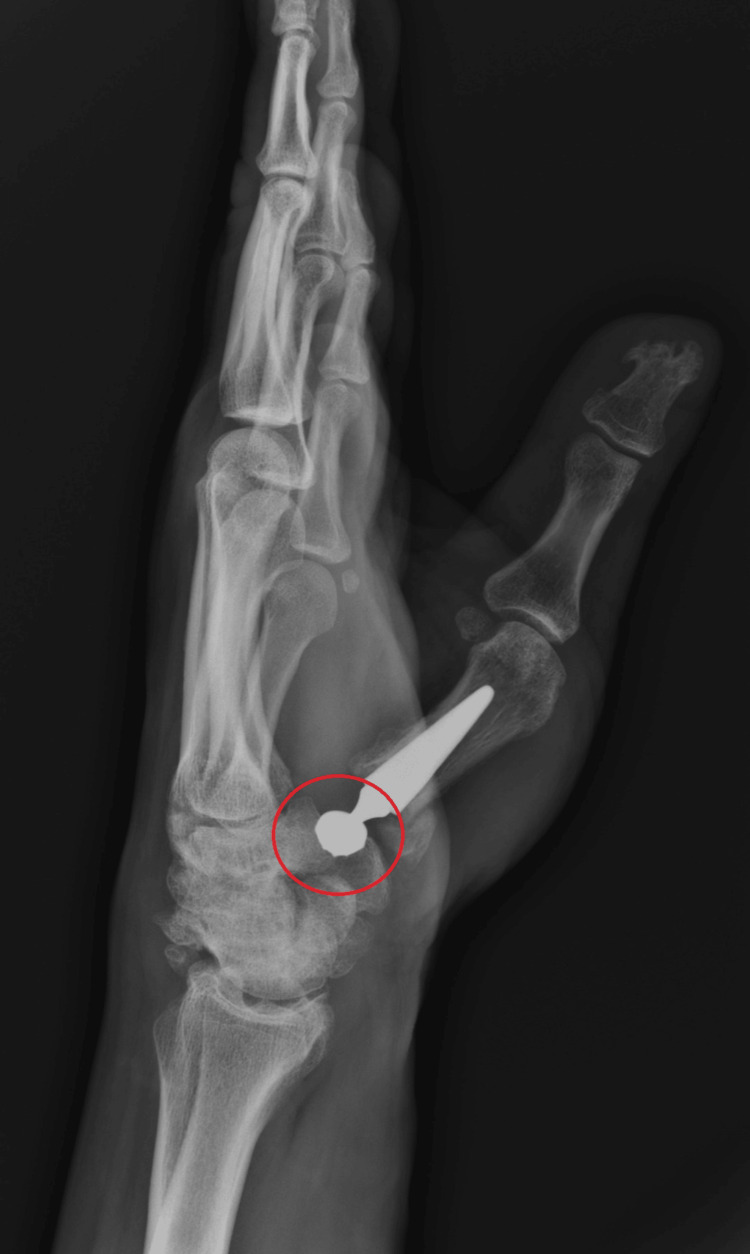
X-ray in the lateral projection obtained two years after surgery The correct positioning of all endoprosthesis elements (head of a double mobile CMC thumb prosthesis and metal acetabular shell) is marked in red. CMC: carpometacarpal
